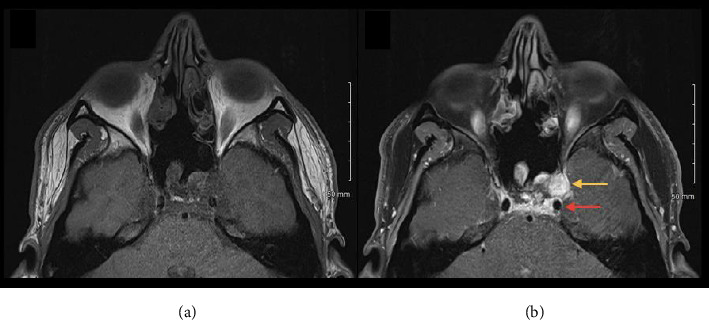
MRI brain (a) pre- and (b) postcontrast demonstrating a contrast-enhancing lesion (yellow arrow) medial to the left temporal lobe within the cavernous sinus, and anterior to the internal carotid artery (red arrow).
